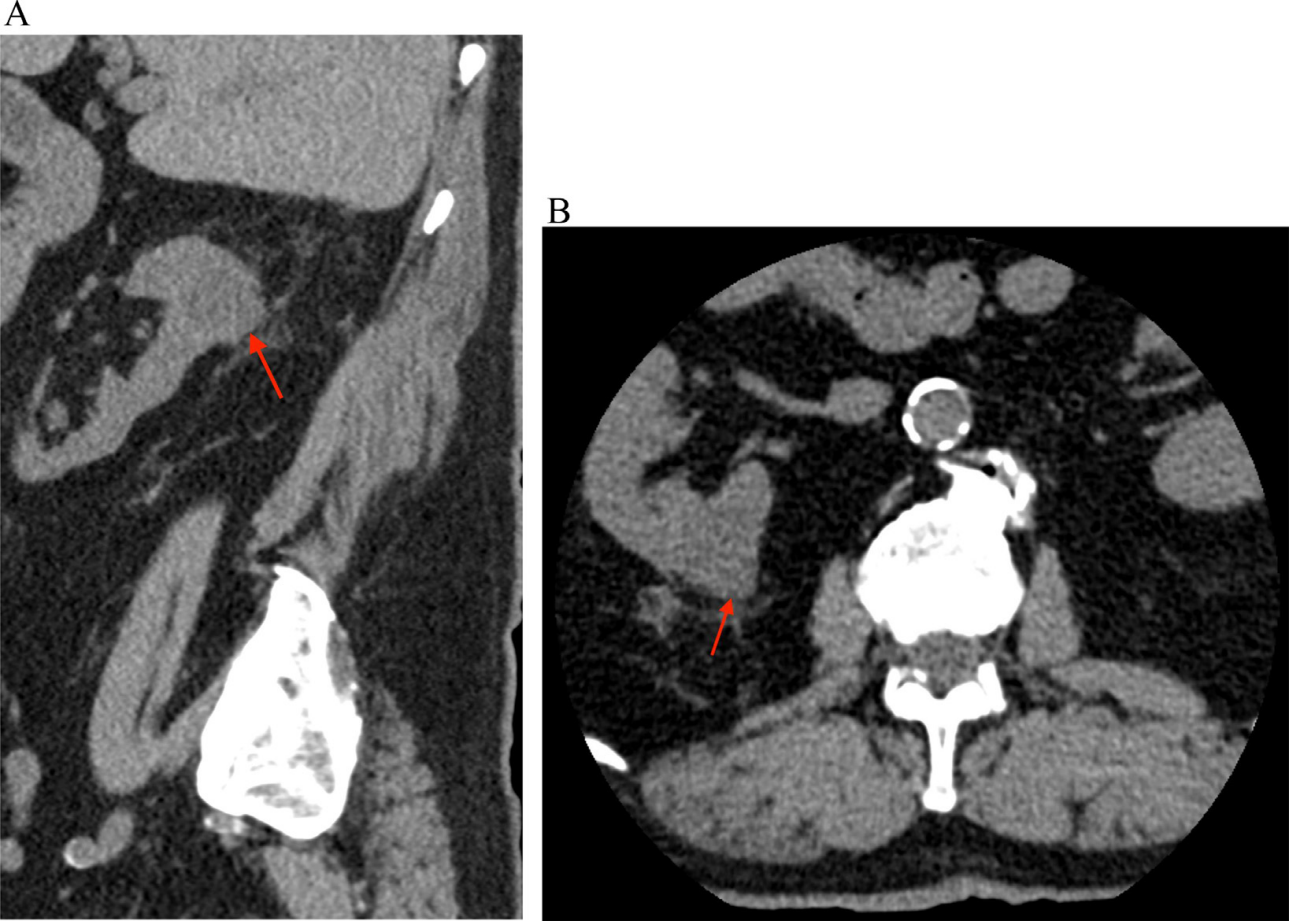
A noncontrast CT image of the lumbar spine. (A) Sagittal and (B) axial CT images reveal an exophytic soft tissue density lesion in the posterior upper pole of the right kidney (red arrow) with surrounding fat stranding.
